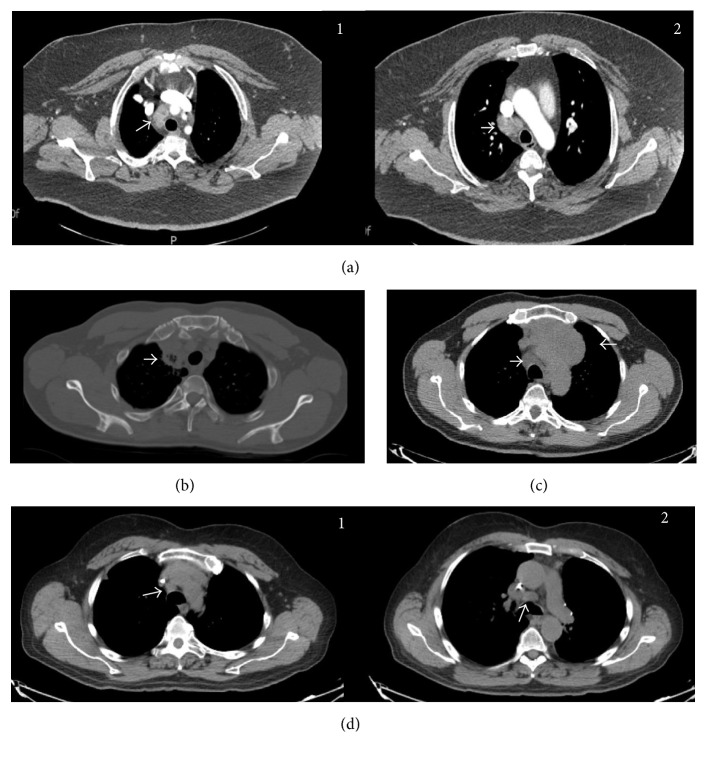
Computed tomography chest scan of lesions compromising station 2R. (a) Right paratracheal adenopathy extending from station 2R white arrow (Panel 1) to the level of 4R white arrow (Panel 2). (b) Right paratracheal/parenchymal mass (white arrow) compromising vascular structures. (c) Anterior mediastinal mass with pathologic 4R adenopathy (white arrows). Mass extends to the level of 2R. (d) Adenopathy station 2R (Panel 1) and 4R (white arrows) (Panel 2).
